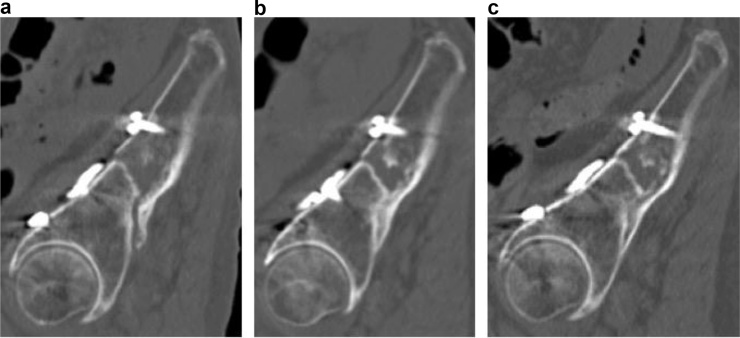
Post-operative computed tomography sagittal views. Computed tomography images showing the process of union of the fracture site at (a) 5, (b) 12 and (c) 19 months after surgery reveal that union occurred between 12 and 19 months post-operatively.
